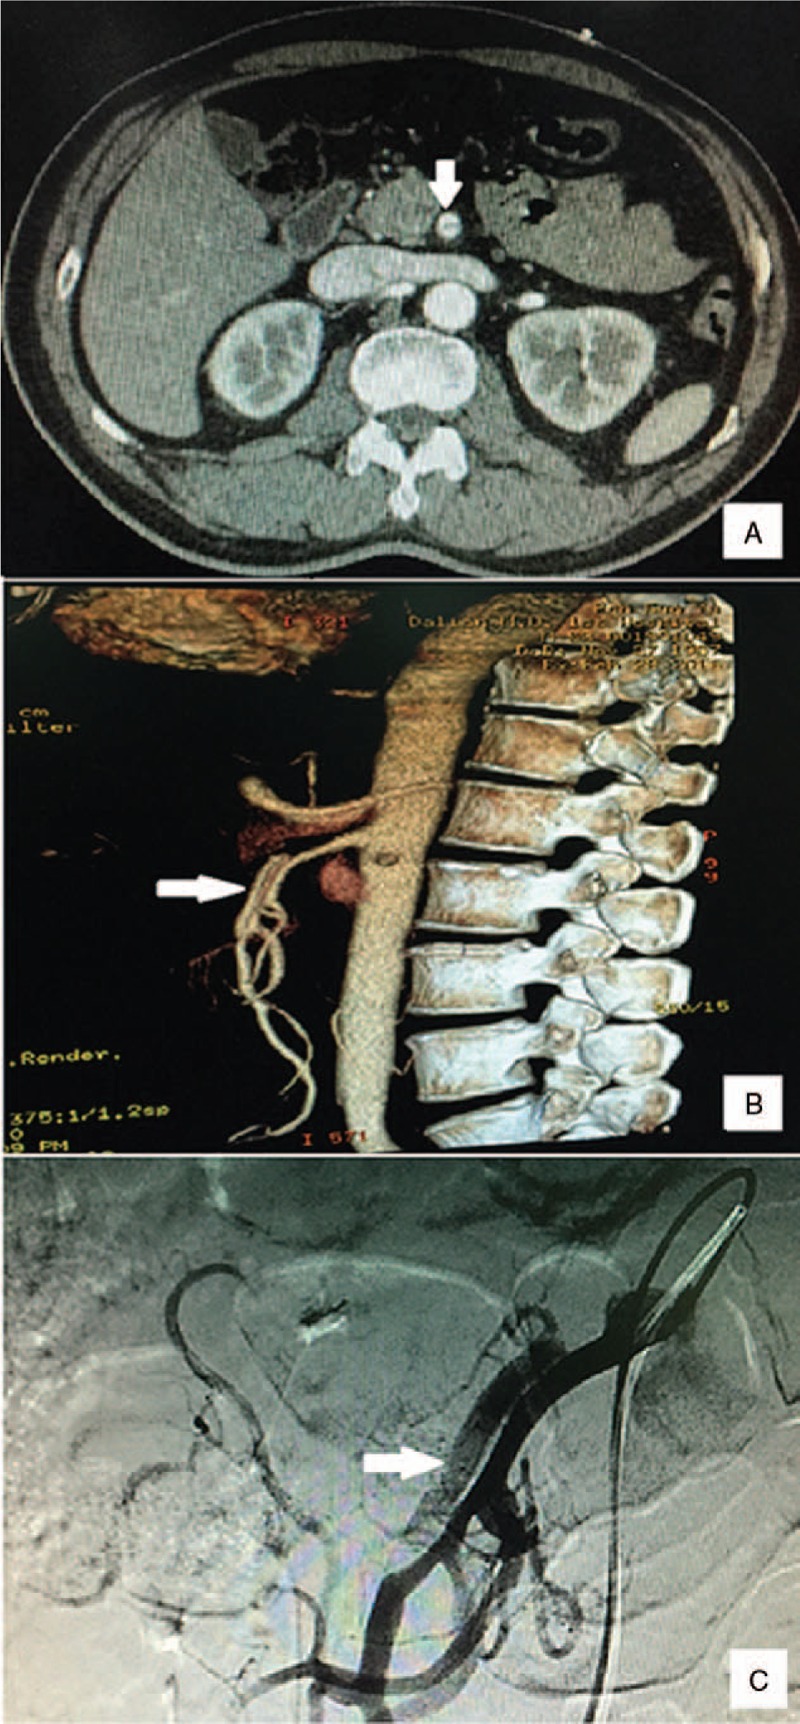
Diagnosis of spontaneous isolated dissection of the superior mesenteric artery. The characteristic finding of a “double lumen sign” of the superior mesenteric artery was found on axial views of computed tomography images (A), and a dissected segment of superior mesenteric artery was found on computed tomography angiography (B) and selective mesenteric angiography (C).
